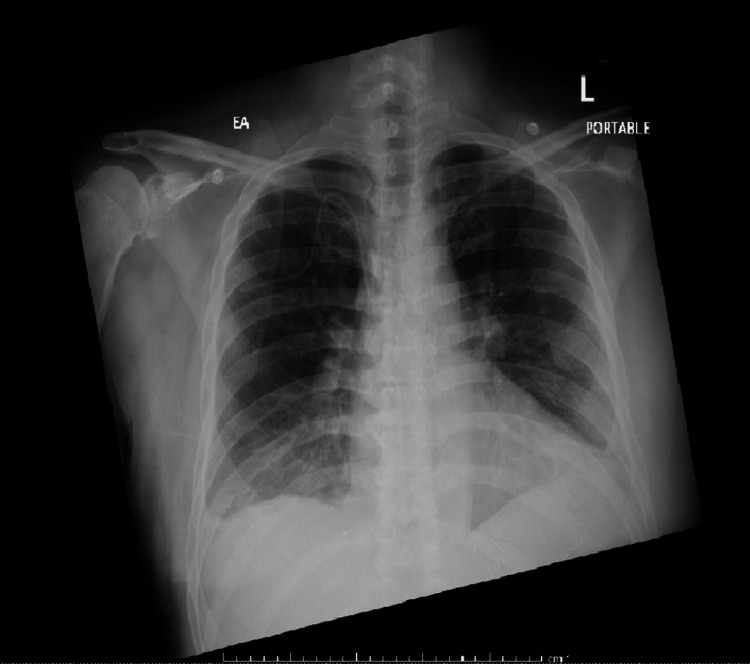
Chest X-ray on the fourth day of ICU stay showing significant improvement
